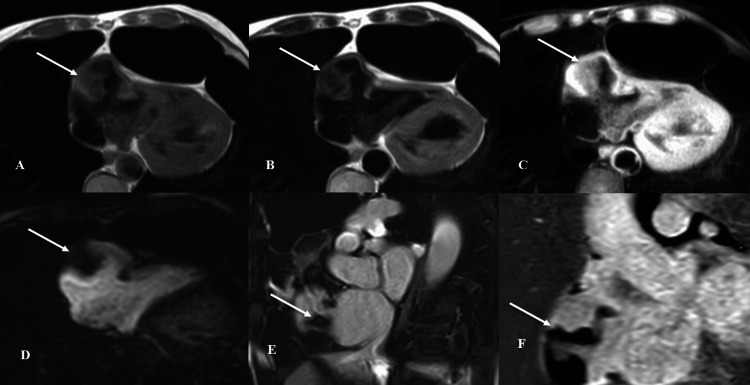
Multi-sequence CMR imaging characterizes the aforementioned lesion. A: T1WIs reveal isointensity (arrow). B: T2WIs depict hypointensity (arrow). C: The absence of signal dropout on FS WIs (arrow). D: No contrast uptake in perfusion images (arrow). E and F: EGE and PSIR images show a dark signal suggesting thrombosis within the lesion (arrow). CMR: cardiac magnetic resonance, TSE: turbo spin echo, EGE: early gadolinium enhancement, PSIR: phase-sensitive inversion recovery, T1WI: T1-weighted image, T2WI: T2-weighted image, FS WI: fat-suppressed image
